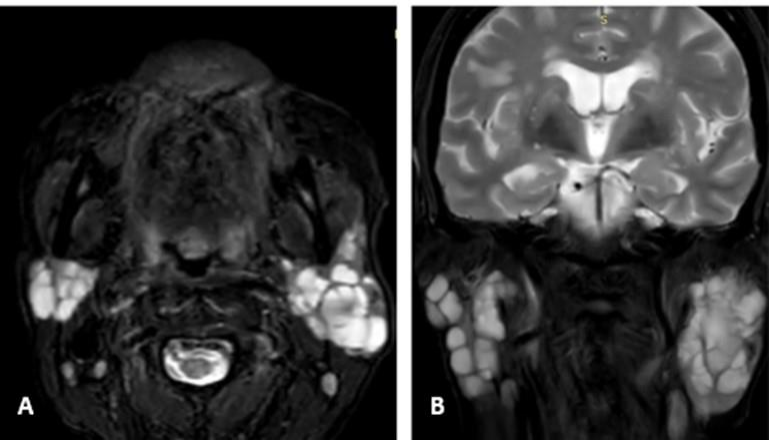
T2-weighted axial (A) and coronal (B) MRI images demonstrating multiple, partially confluent cysts in both parotid glands.
